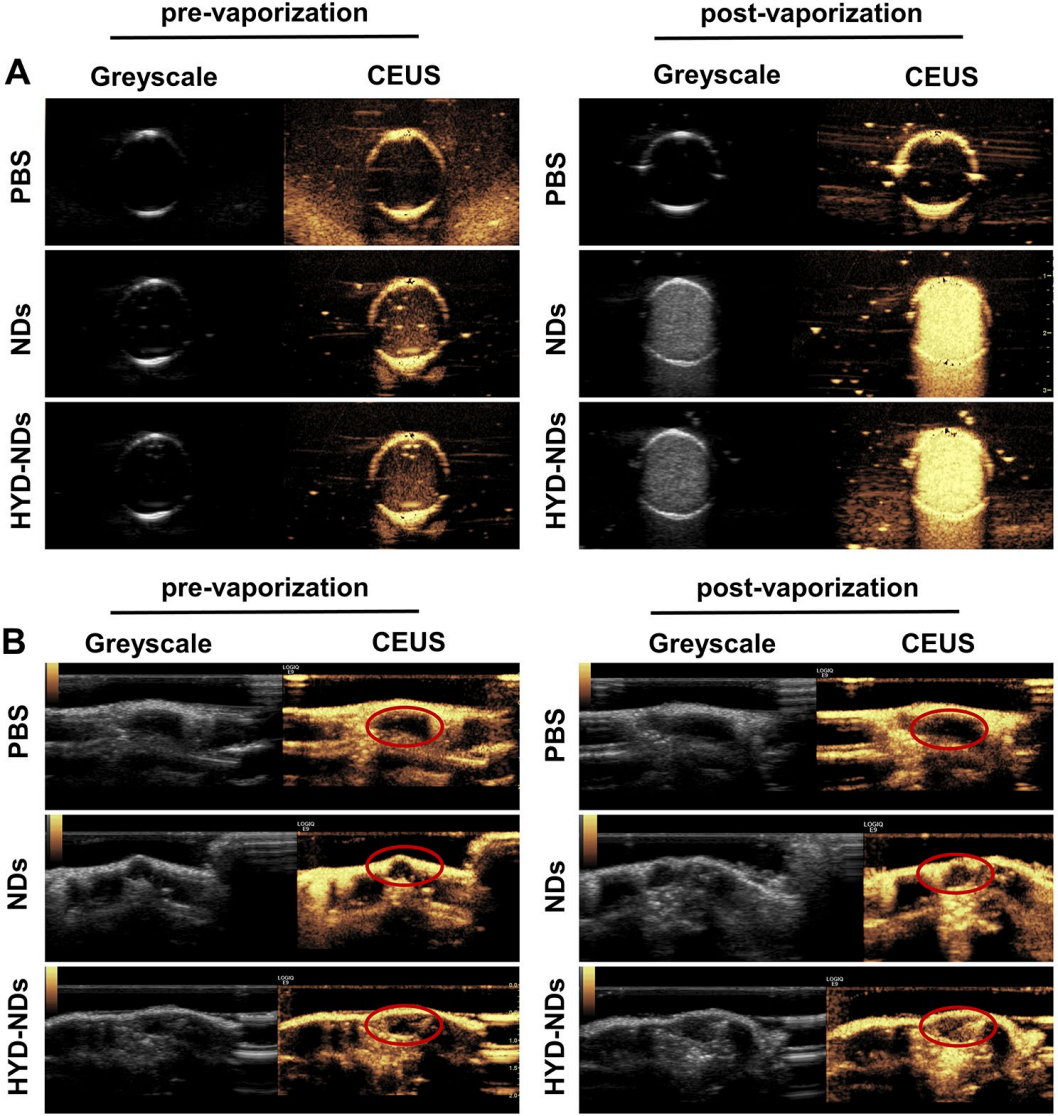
CEUS of NDs and HYD-NDs. (A) Ultrasound imaging in vitro. (B) Ultrasound imaging in vivo. Tumor site shown by red circle
